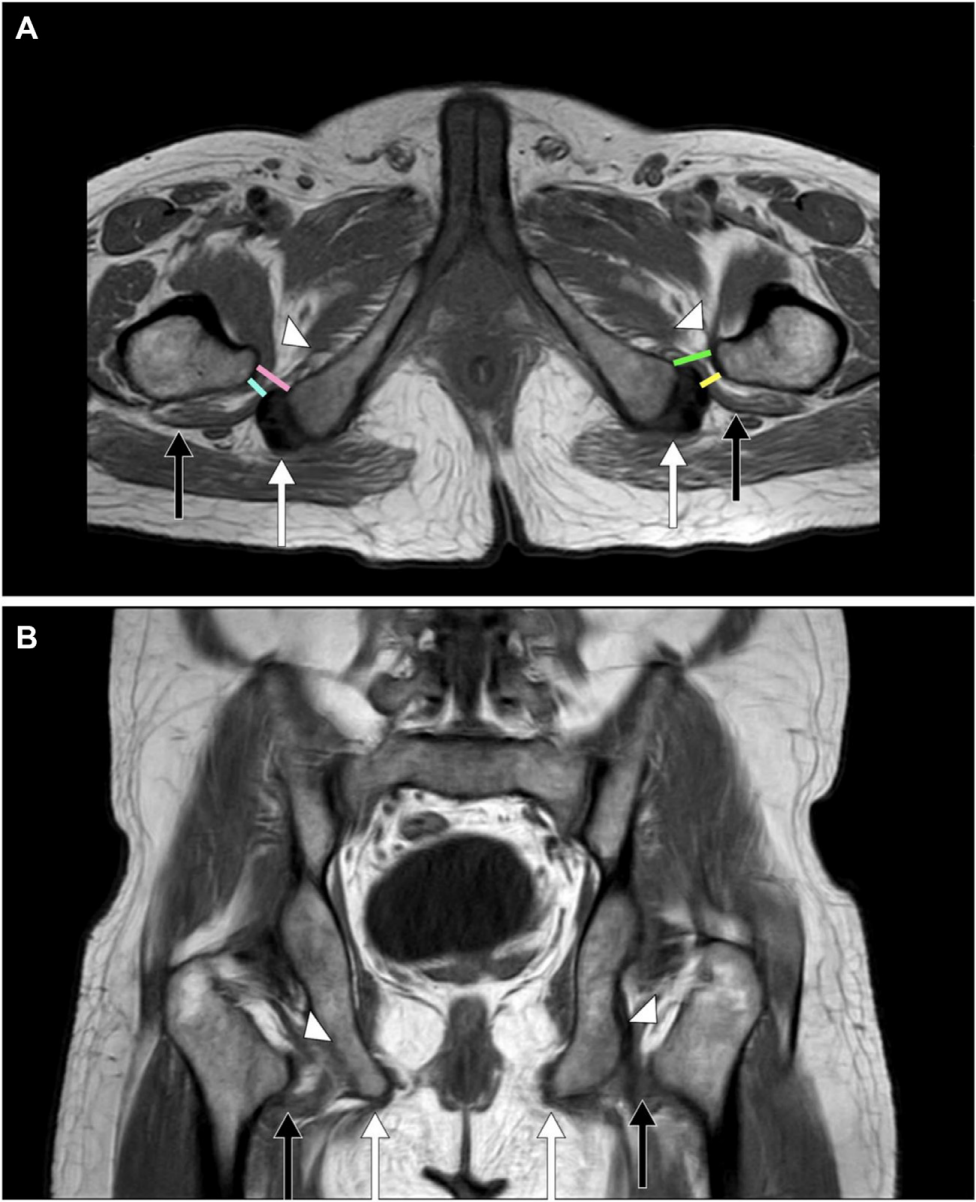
A 33-year-old male presented to the emergency room (ER) with bilateral progressive hip pain for 2 years, which was insidious in onset and persistent even at rest. *Magnetic resonance imaging (MRI) of the hips: T1-weighted (T1W) (A) Axial and (B) Coronal sections* shows a mild decrease in the right ischiofemoral space and quadratus femoris space, measuring 11 mm and 10 mm in the distance respectively and a mild decrease in the left ischiofemoral space and quadratus femoris space, measuring 12 mm and 10 mm in the distance respectively with iliopsoas tendons *(white arrow heads)*, quadratus femoris muscles *(short black arrows),* and hamstring tendons *(short white arrows).* Note: right ischiofemoral space *(pink line),* right quadratus femoris space *(cyan line),* left ischiofemoral space *(green line)*, and left quadratus femoris space *(yellow line).*
